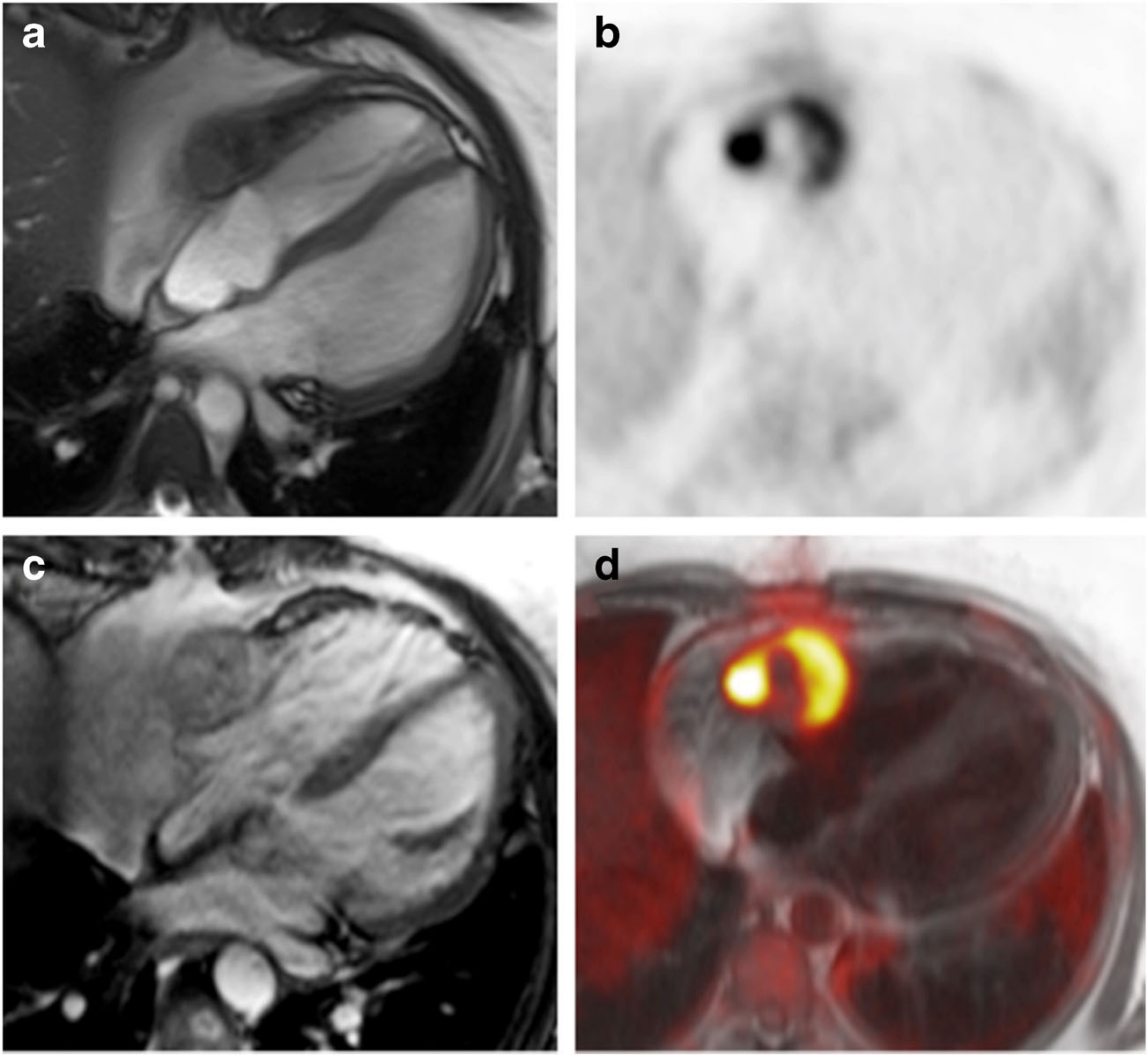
(A) Cine MR image of an angiosarcoma infiltrating the free wall of the right ventricle and atrium with adjacent pericardial effusion. (B) ^18^F-FDG PET shows intense but heterogeneous ^18^F-FDG uptake within the tumour and otherwise suppressed myocardial ^18^F-FDG uptake by the use of a high-fat low-carbohydrate protein-permitted diet. (C) The tumour demonstrates heterogeneous and overall moderate enhancement on T1-weighted MRI after intravenous application of gadolinium-based contrast agent. (D) Fused images show excellent spatial agreement between PET and MRI
